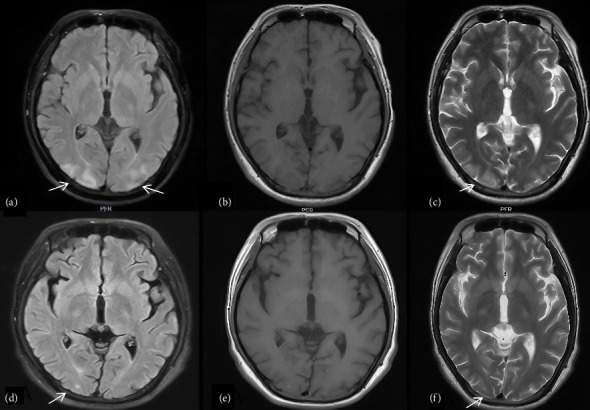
(a) Cranial MRI showed diffuse symmetrical patchy FLAIR with high signals (arrows) in the bilateral parieto-occipital cortex and bilateral cerebellar hemispheres, (b) equal or low signal in T1WI, (c) high signal in T2WI, and (d, e, f) the abnormalities seen in FLAIR, T1WI, and T2WI had essentially disappeared in a cranial MRI performed 18 d later.
